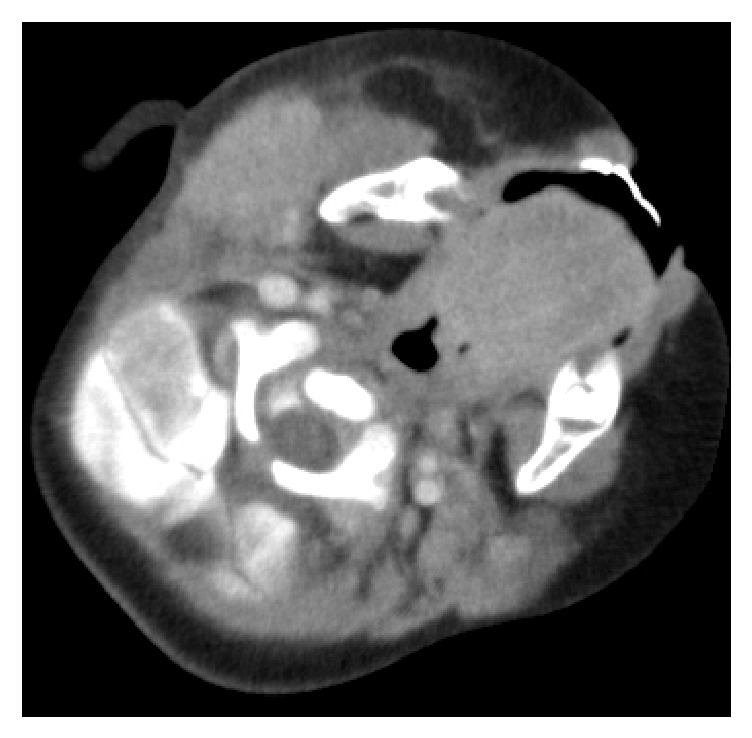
An enhanced computed tomography scan of the pharynx showed increased intensity in the right parotid grand.
